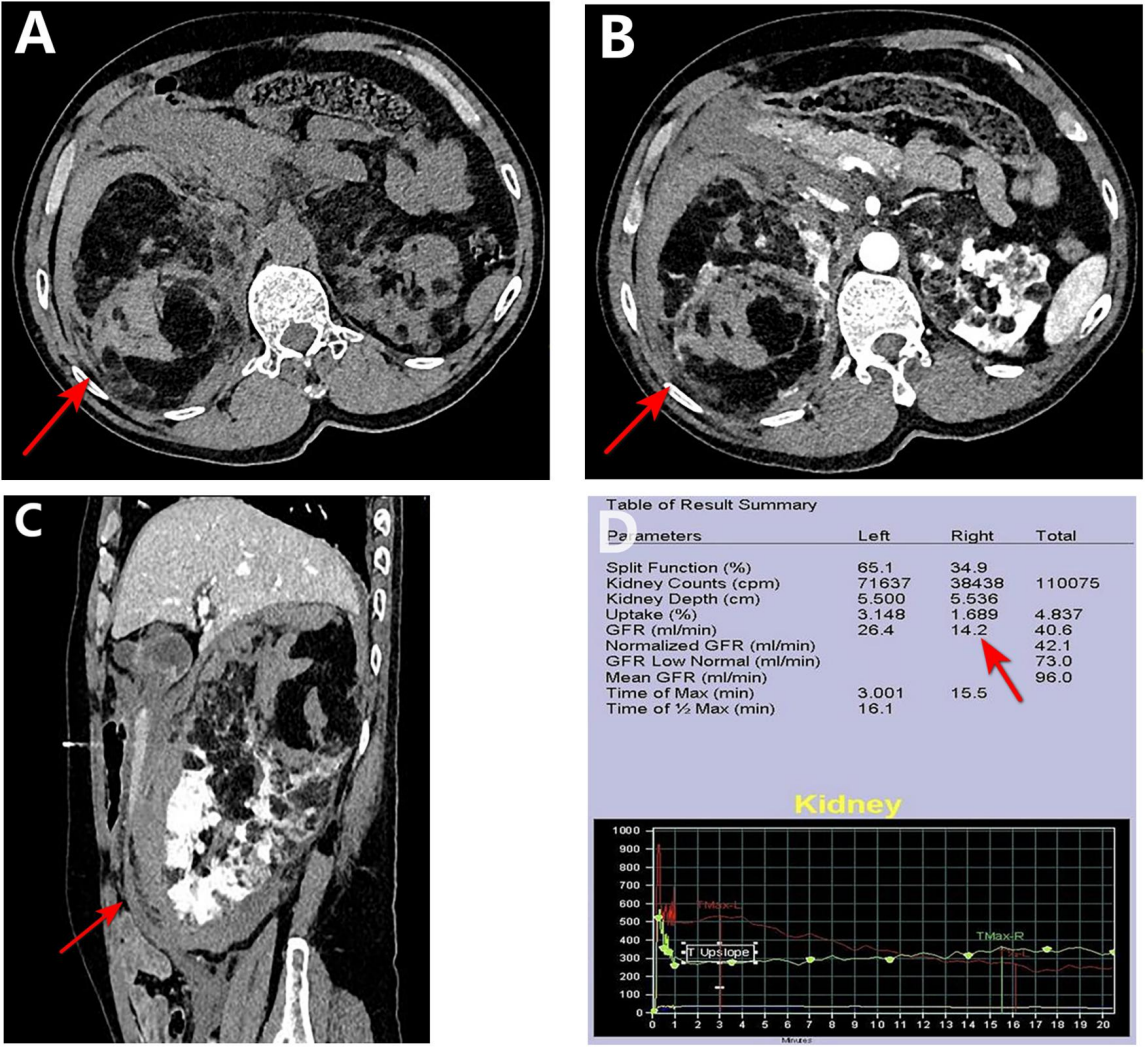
Preoperative CT and renal function. (A–C) CT images show a ∼17.5 cm mass (arrows) in the right kidney with hemorrhage and poor enhancement. (D) GFR measurement confirms markedly reduced right kidney function.
